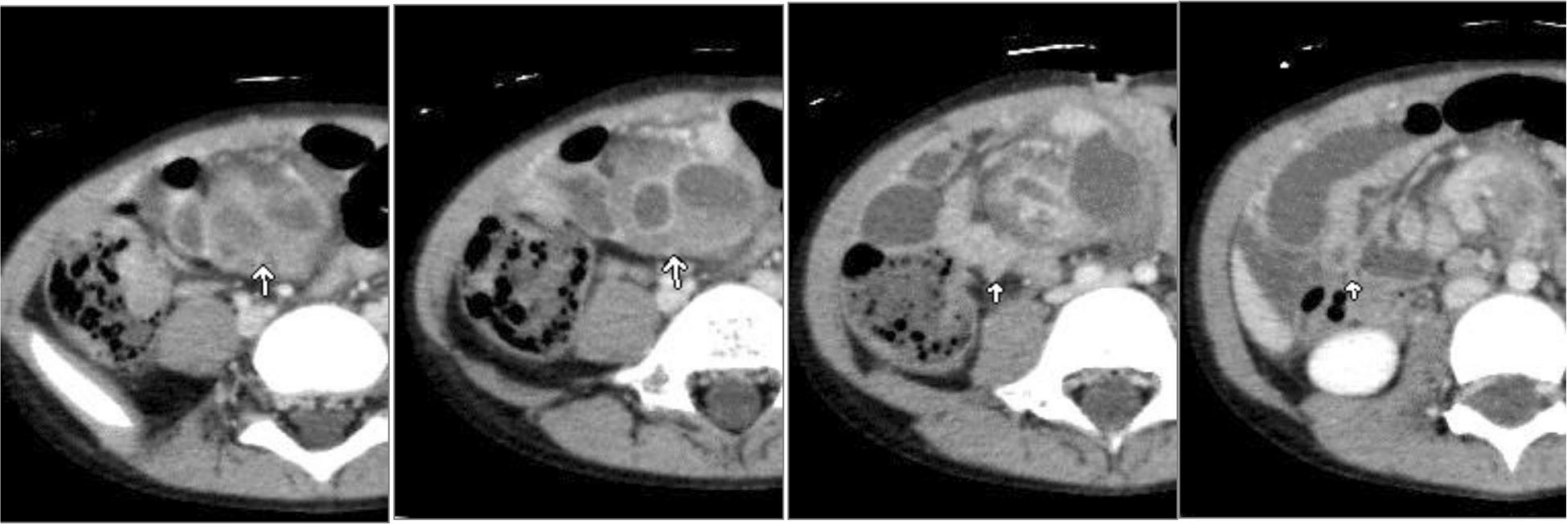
Enhanced CT, retrospective finding. An independent blood vessel coursing through the dorsal side into the lesion was identified (arrows)
